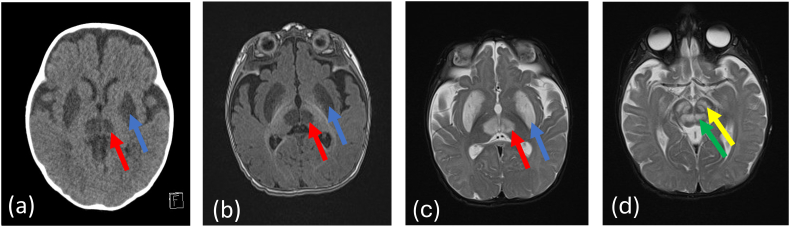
(a) Axial CT and (b) MRI T1 weighted imaging showing hypointensity of the putamina (blue arrows) and thalami (red arrows); (c) T2 hyperintensity of the putamina (blue arrows) and thalami (red arrows); (d) T2 showing midbrain lesions in the substantia nigra (yellow arrow) and red nuclei (green arrow).
